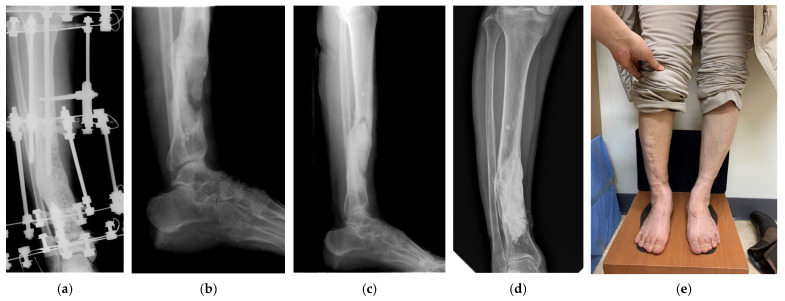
Antibiotic-mixed cement filling in a 41-year-old female patient (identified pathogen: Burkholderia cepacia, Bacteroides species, and Prevotella species). (a) Anteroposterior radiograph shows external fixation and antibiotic-impregnated cement bead insertion for chronic osteomyelitis of the distal tibia shaft in the previous hospital. (b) The lateral radiograph shows the removal of the external fixator and antibiotic-impregnated cement bead. (c) Lateral radiograph shows antibiotic-impregnated cement filling into the tibia shaft 2 weeks postoperatively. (d) Anteroposterior radiograph shows antibiotic-impregnated cement filling is well maintained 16 years postoperatively. (e) Medical photo shows bowing leg and no recurrence of infection 16 years postoperatively.
